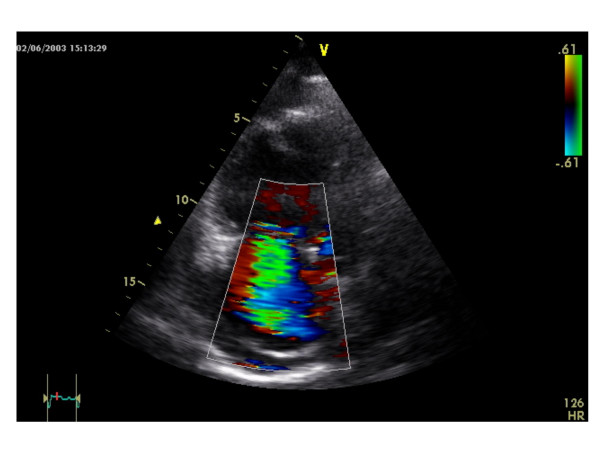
Tricuspid insufficiency grade II–III with a morphological normal valve at initial investigation with transthoracic echocardiography.
